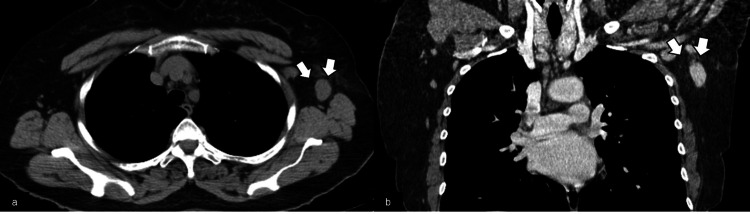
Multidetector computed tomography (MDCT). The axial (a) and the coronal (b) MDCT demonstrate axillary lymphadenopathy in the left (arrows).
